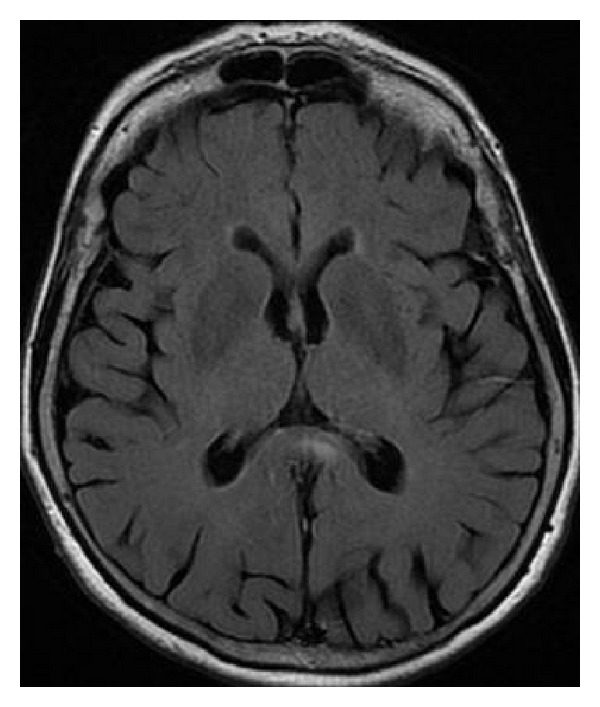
FLAIR, axial plane. Old isolated infarct in the callosal splenium.
